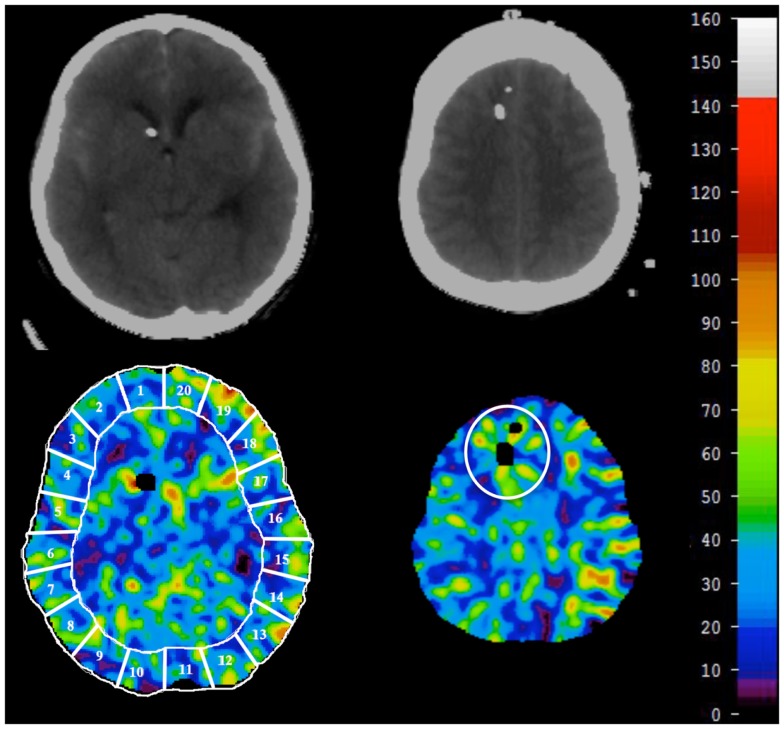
At each level, CBF in 20 ROIs was calculated and averaged for global CBF (lower left). The vascular territories were analyzed as follows: anterior cerebral artery ROI 1–2 (lower right) and 19–20 (lower left), middle cerebral artery ROI 3–8 (lower right) and 13–18 (lower left), and posterior cerebral artery ROI 9–10 (lower right) and 11–12 (lower left). For CBF around the microdialysis catheter, a ROI was manually drawn around the microdialysis catheter after localization on CT-scan (lower right).
